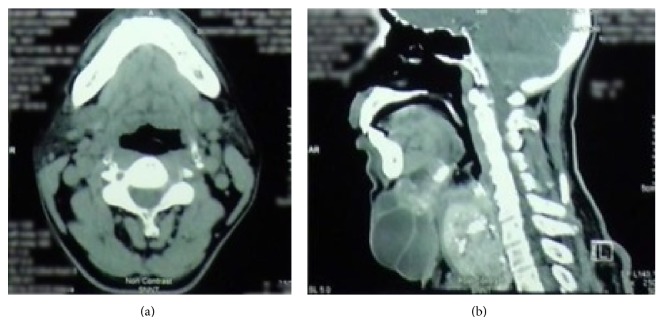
Axial and transverse section of a CT of the neck.
